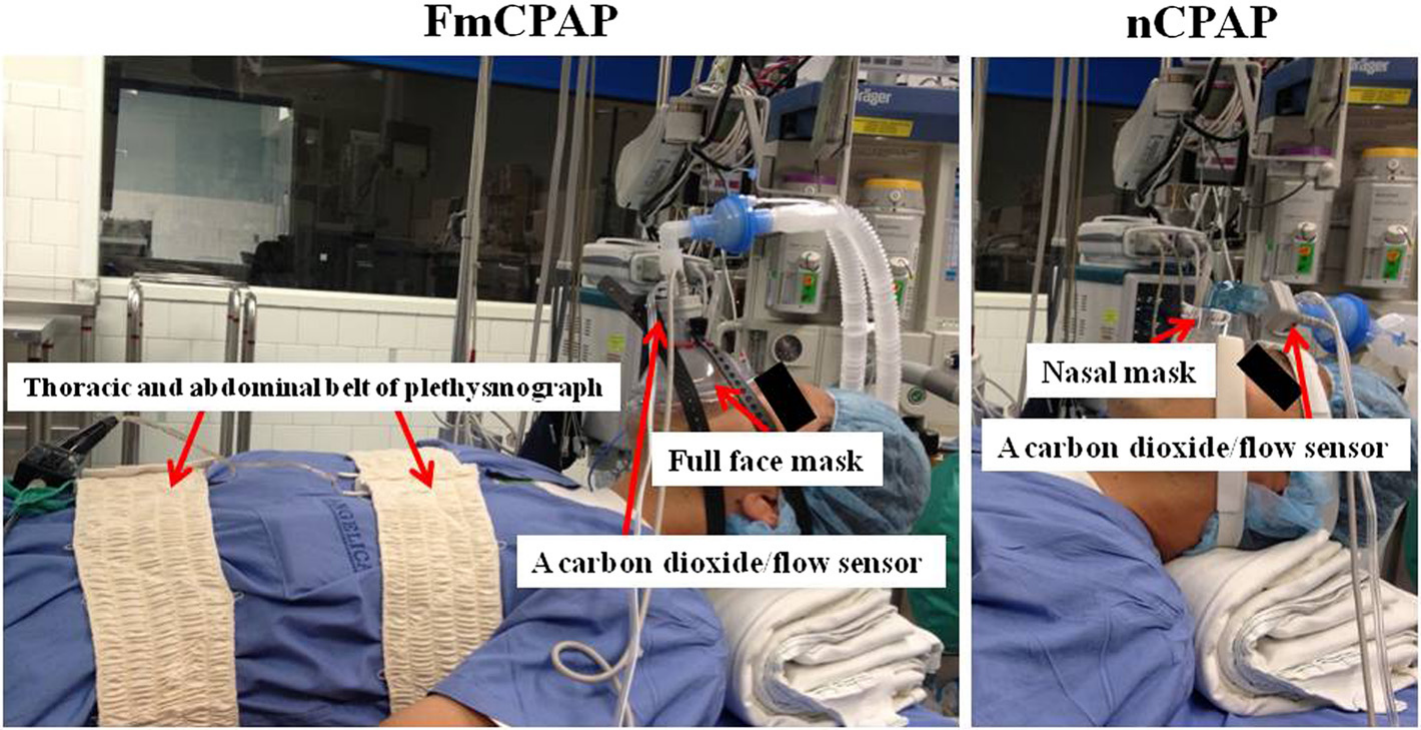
Experiment set-up. Left: continuous positive airway pressure via a full face mask and ventilation (FmCPAP). A carbon dioxide (CO_2_)/flow sensor (Novametrix Medical Systems Inc., Wallingford, CT, USA) was placed between the mask and the breathing circuit. The sensor was connected to a non-invasive cardiac output (NICO) monitor (model 7300; Respironics, Murrysville, PA, USA). This sensor allows continual monitoring and recording of the following data: flow waveform, airway pressure, respiratory rate, tidal volume, and exhaled CO_2_ waveform. With the respiratory inductance plethysmograph (Respitrace Calibrator; Ambulatory Monitoring, Inc., Ardsley, NY, USA), the rib cage band was placed at the level of the nipples and the abdominal band at the level of the upper abdomen. Right: continuous positive airway pressure via a nasal mask and ventilation (nCPAP). The equipment is the same as with FmCPAP, except for the nasal mask.
